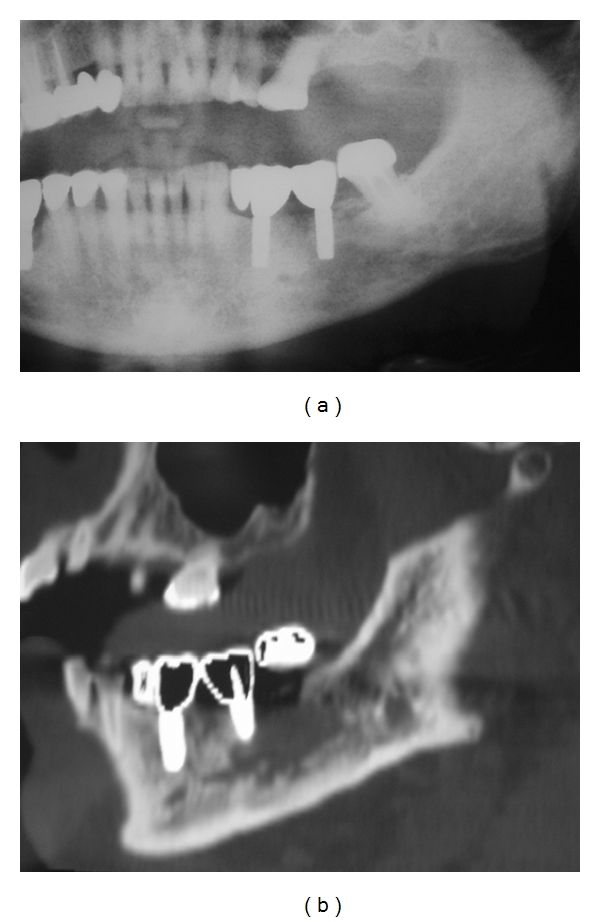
(a) A panoramic radiography showing only low-diffusion radiolucency around the left implant. (b) Computerized tomography image revealing necrotic bone involving left mandibular implant (September 2008).
